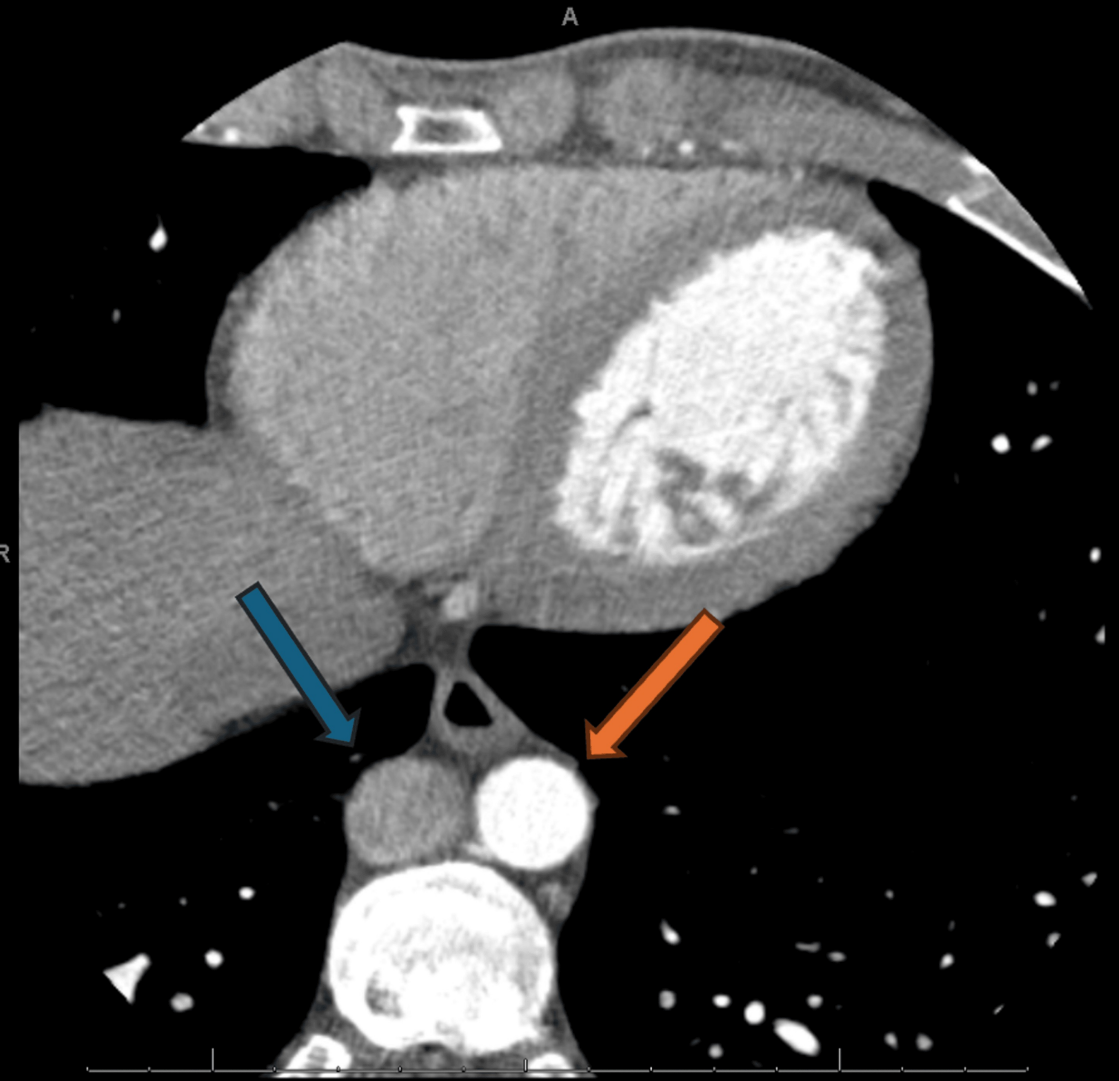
CT coronary angiogram: axial view at the thorax level, showing the continuation of the azygous vein The blue arrow shows the continuation of the azygous vein at the thoracic level. The orange arrow shows the descending aorta for reference. These imaging findings were critical in guiding the decision to attempt a retrograde aortic approach for ablation, as the abnormal venous anatomy complicated standard access.
